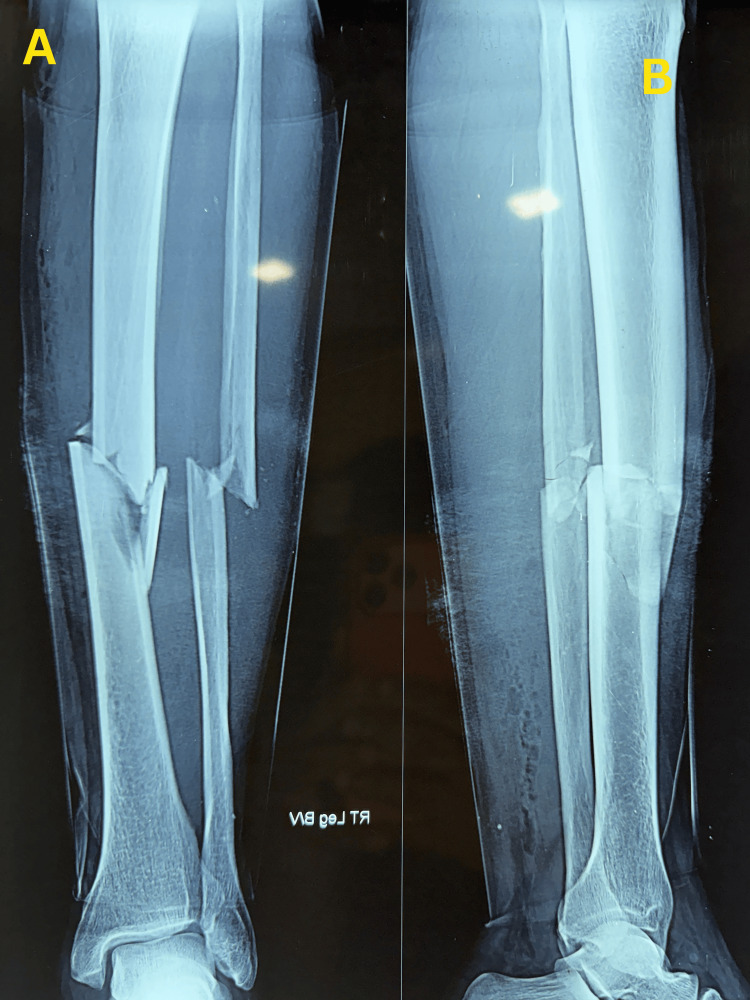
Preoperative radiographs of the right tibial fracture (A) Anteroposterior radiograph showing a comminuted midshaft fracture of the right tibia.
